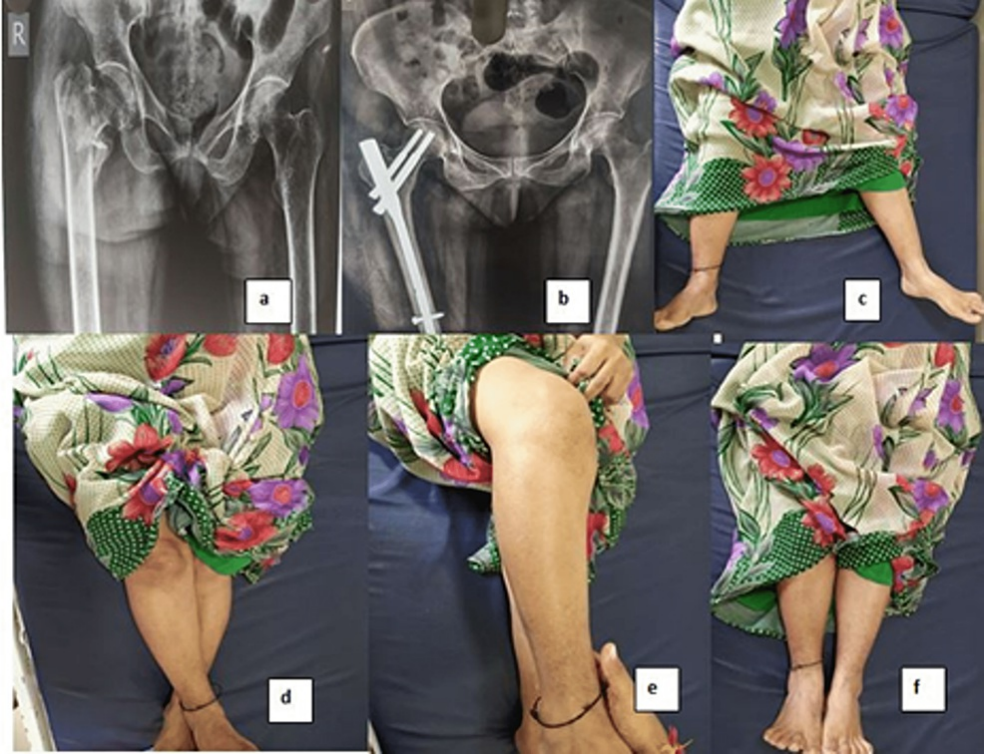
Images of patients showing excellent results A: trauma radiograph, B: union radiograph, C: abduction, D: adduction, E: flexion, F: no limb length discrepancy (pelvis squared)
